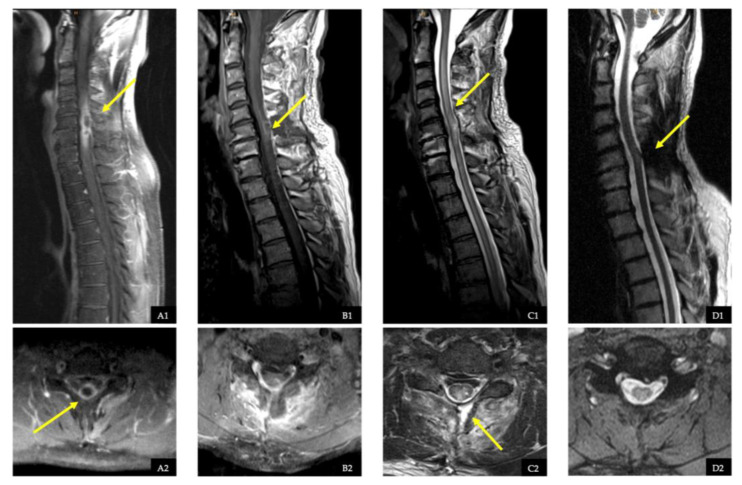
Clinical management of spinal intradural abscess. (A1,A2): Preoperative sagittal and axial contrast-enhanced T1-weighted MR images of the cervical spine showing a spinal intradural abscess (SIA) on the dorsal part of the spinal cord at the level of C5 to C7. The yellow arrows indicate the SIA. (B1,B2): Immediate postoperative sagittal and axial T1-weighted contrast-enhanced MR images of the cervical spine showing postoperative contrast enhancement at the level of C6 (yellow arrow). (C1,C2): Immediate postoperative sagittal and axial T2-weighted MR images showing the postoperative intramedullary hyperintense signal (sign of myelopathy, yellow arrow in C1). The yellow arrow in C2 points out the approach. (D1,D2): Sagittal and axial T2-weighted MR images after 12 weeks at the time of discontinuation of antibiotics, showing no signs of infection.
